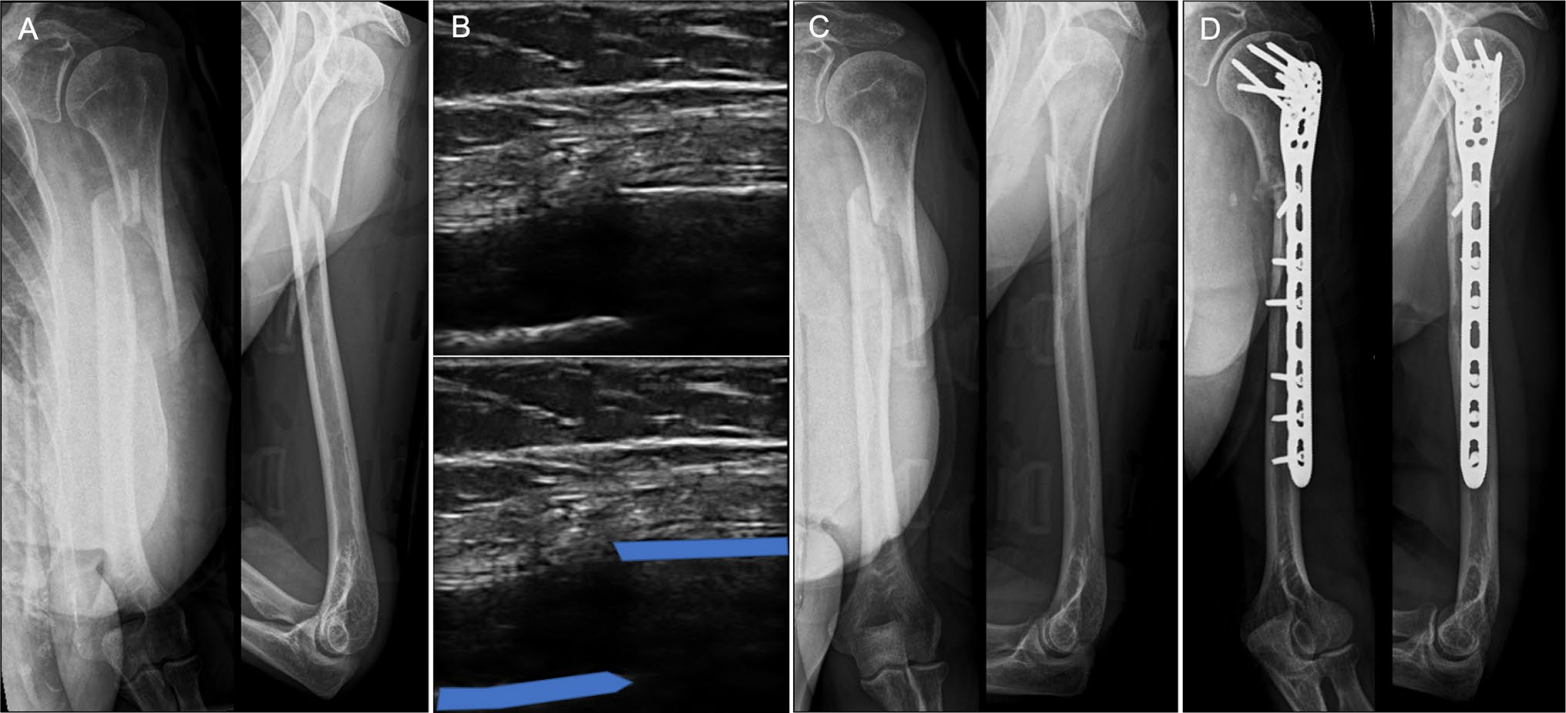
Sequence of images for a 65 year-old female patient with a left humeral shaft fracture: A Six-week anteroposterior (AP) and lateral radiographs (Radiographic Union Score for HUmeral fractures = 6); B Six-week ultrasound scan image (blue = bone cortex); C 12-week AP and lateral radiographs (showing nonunion); D AP and lateral radiographs following nonunion surgery (performed at 37-weeks post-injury)
